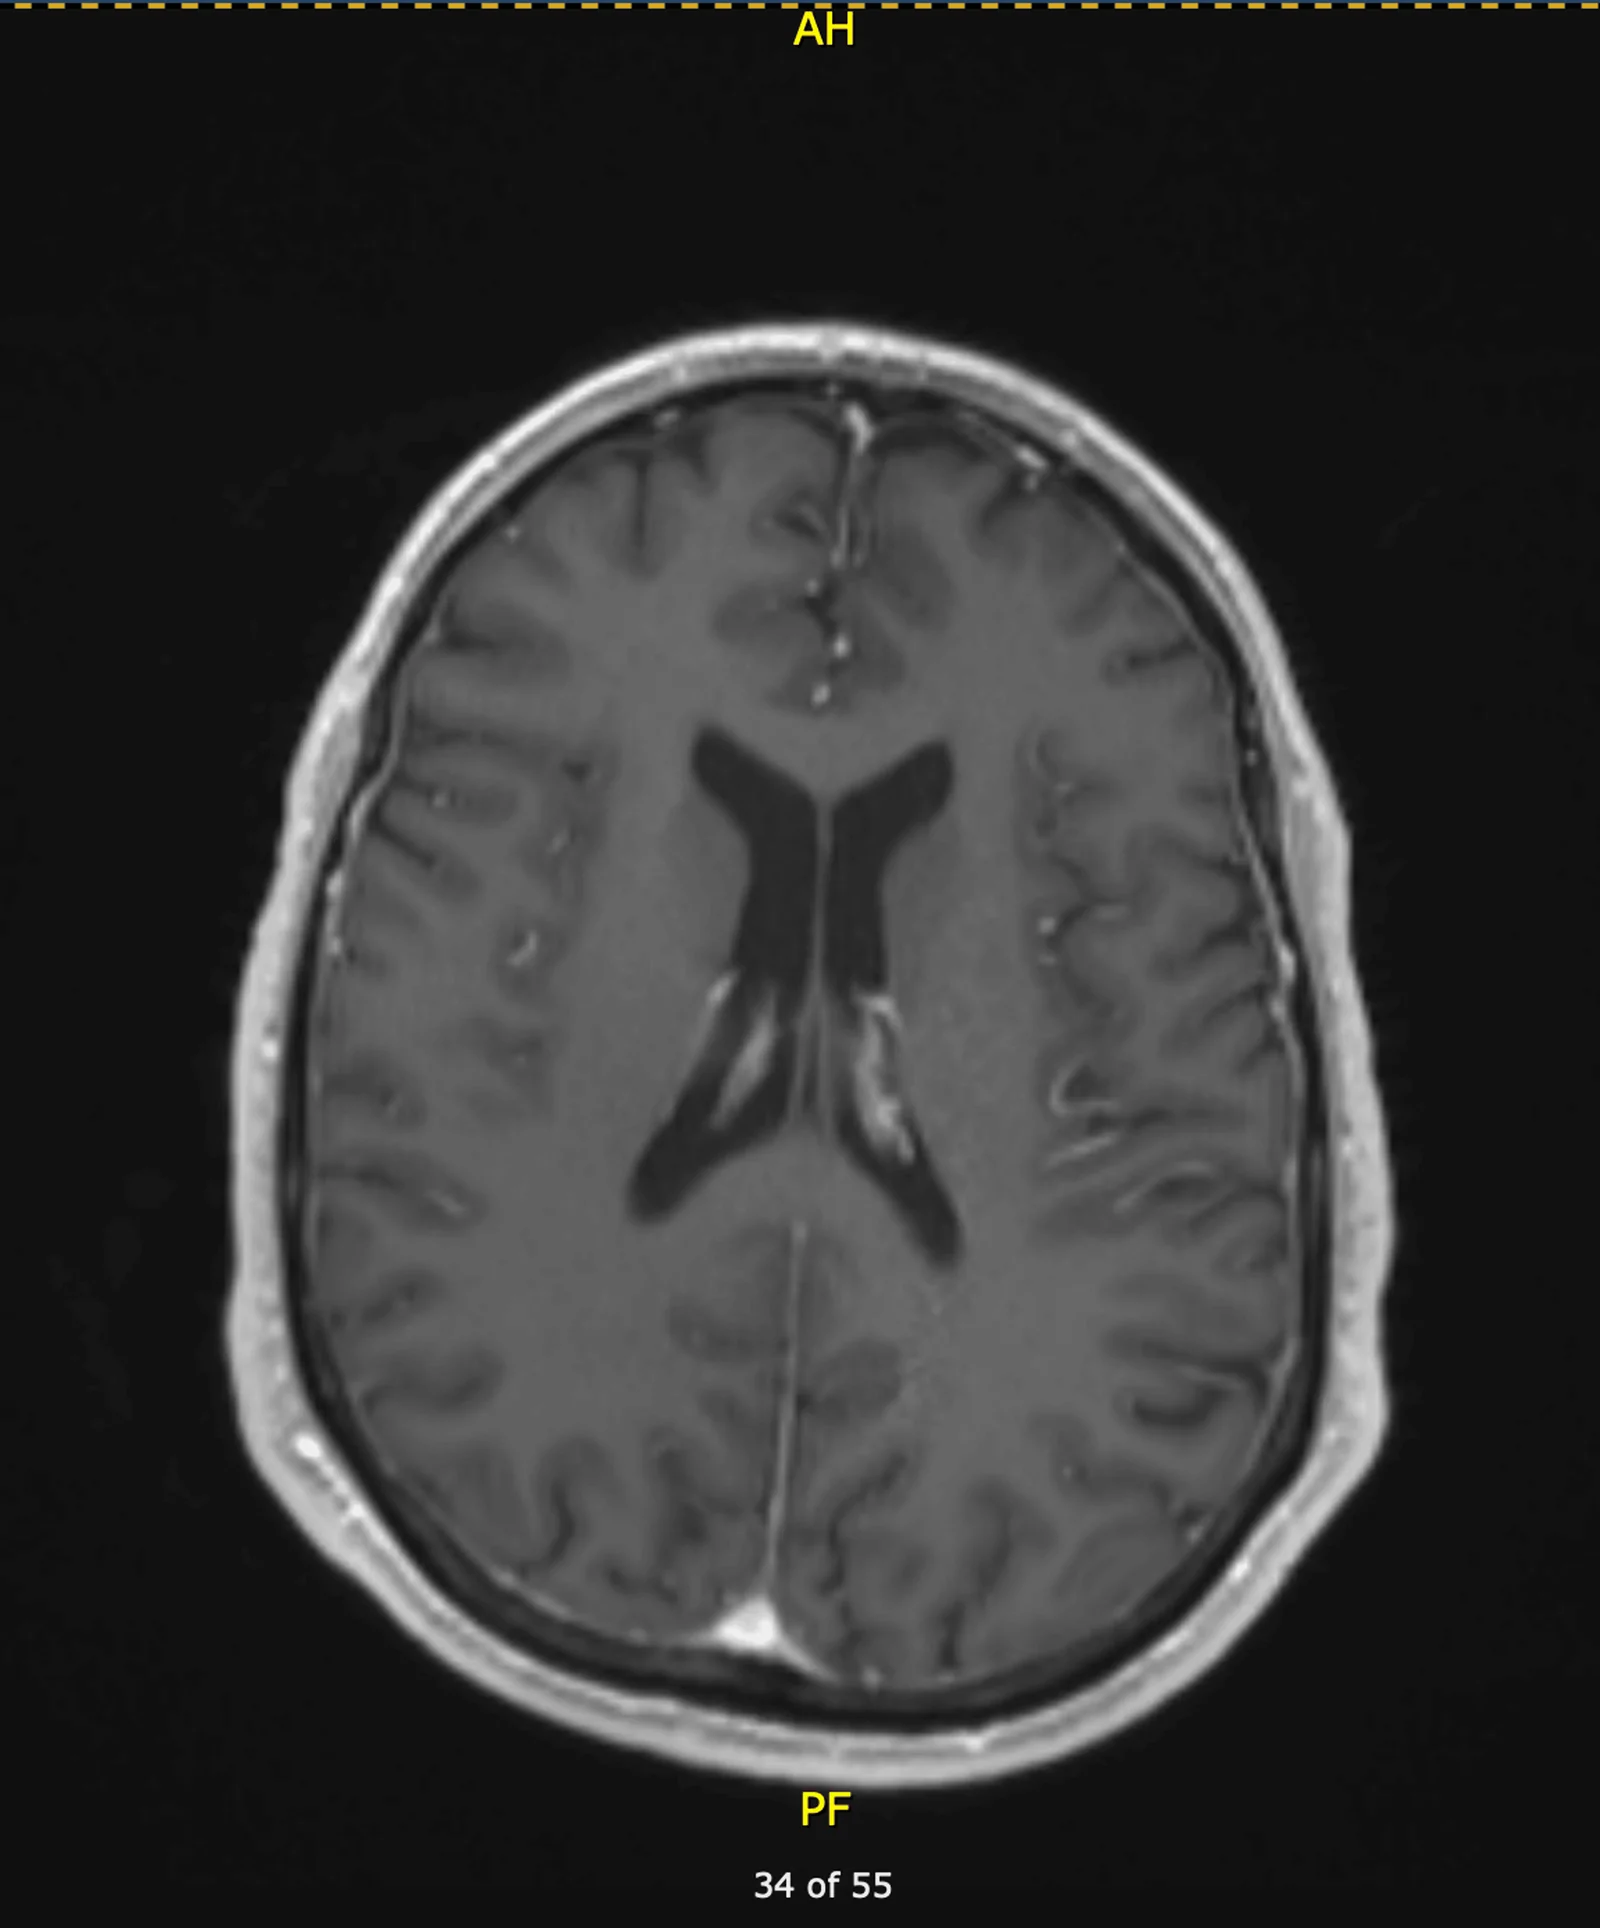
Axial post-contrast T1-weighted MRI brain demonstrating no acute intracranial hemorrhage, mass effect, midline shift, or intracranial metastasis.
